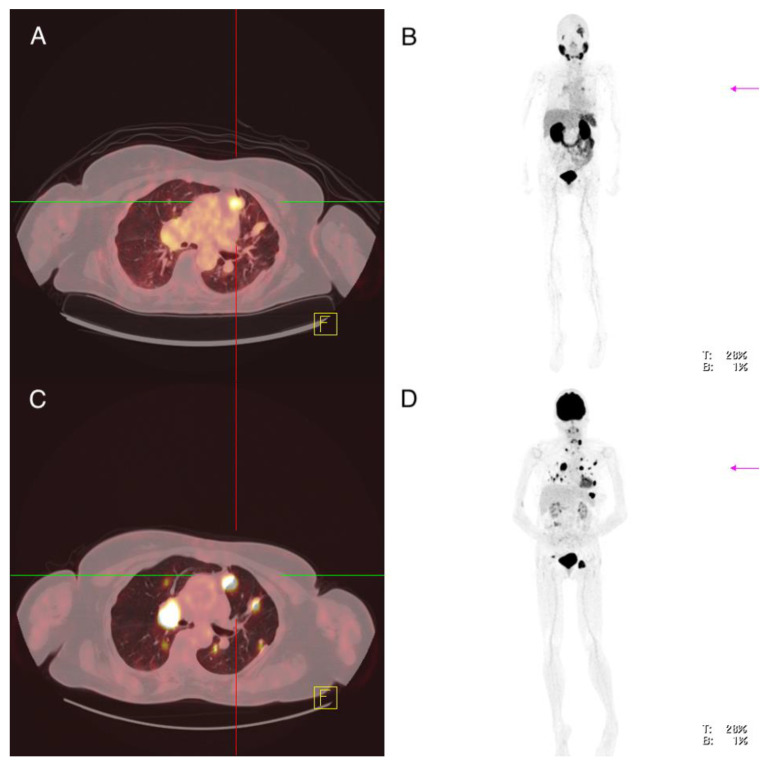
Patient 7: ^68^Ga-PSMA-PET/CT (A,B), ^18^FDG-PET/CT (C,D). Inhomogeneous PSMA expression of mediastinal lymph node metastases in the absence of PSMA expression of pulmonary lesions with increased glucose uptake.
